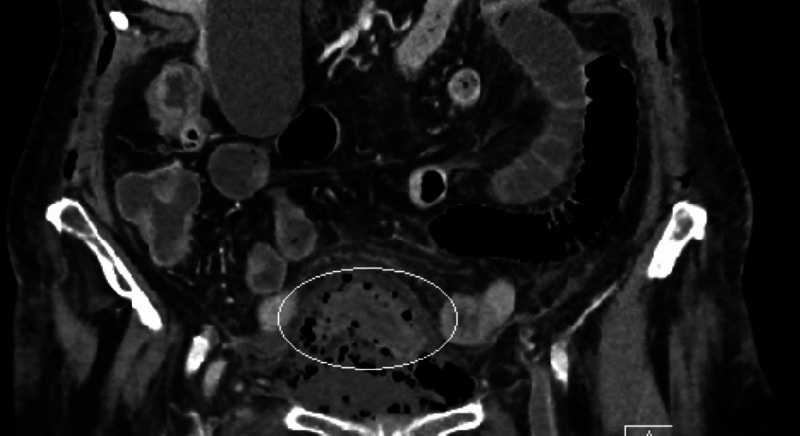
CT scan – coronal view – the gas from the periappendiceal abscess encircles the entire urinary bladder
